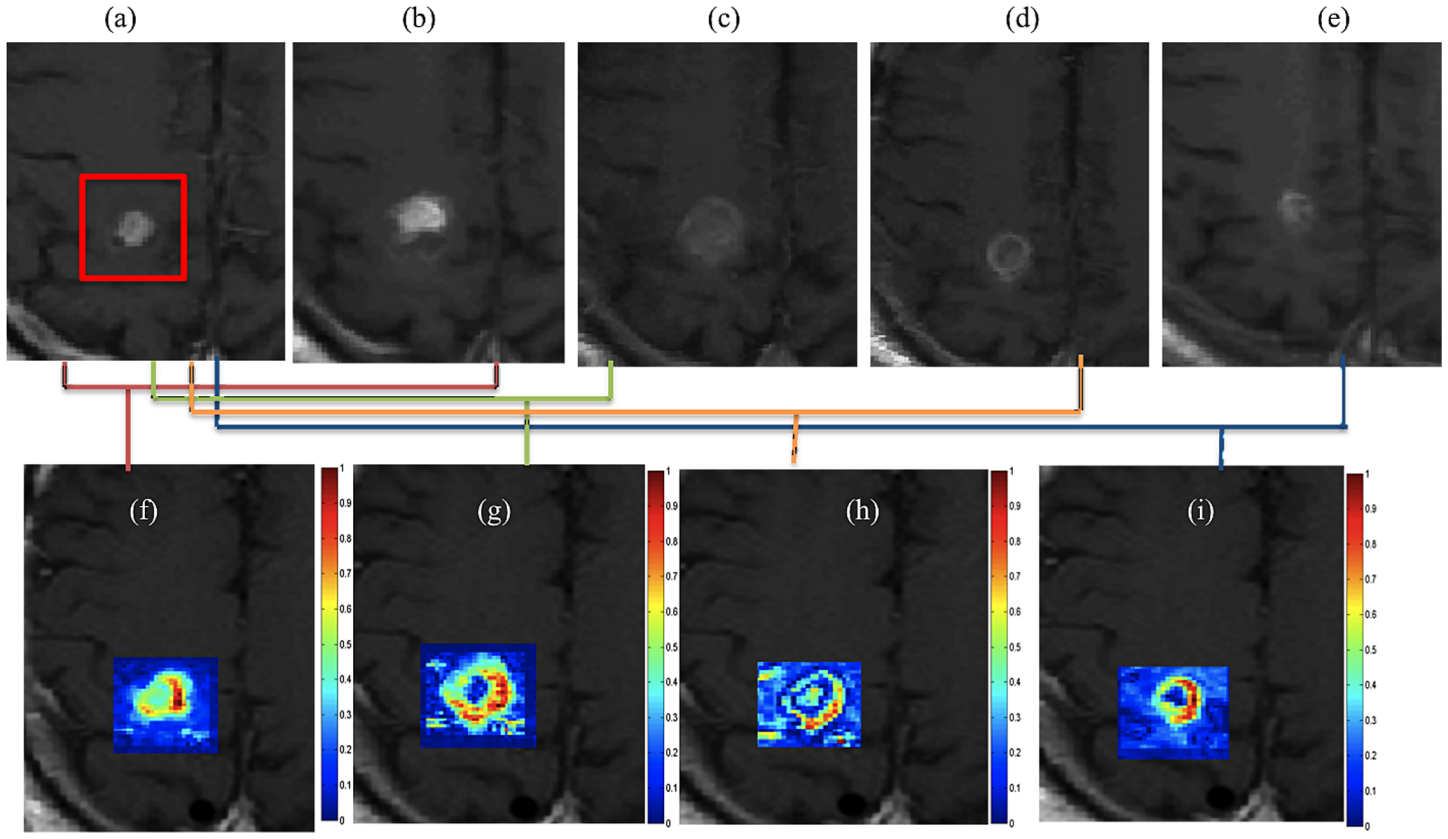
Original T2-w MRI images for (a) baseline (pre-LITT), (b) 24-hour, (c) 1-month, (d) 3-month, (e) 6 month post-LITT. Figs. 6(f)
